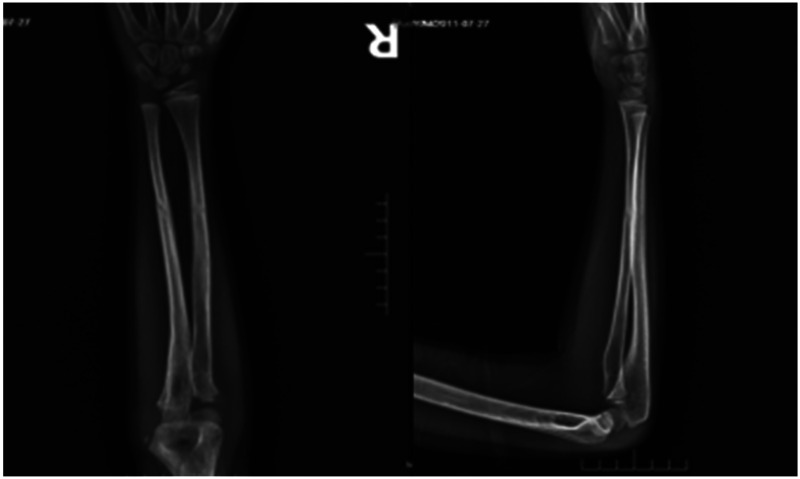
X-rays after 5 months of reset.
